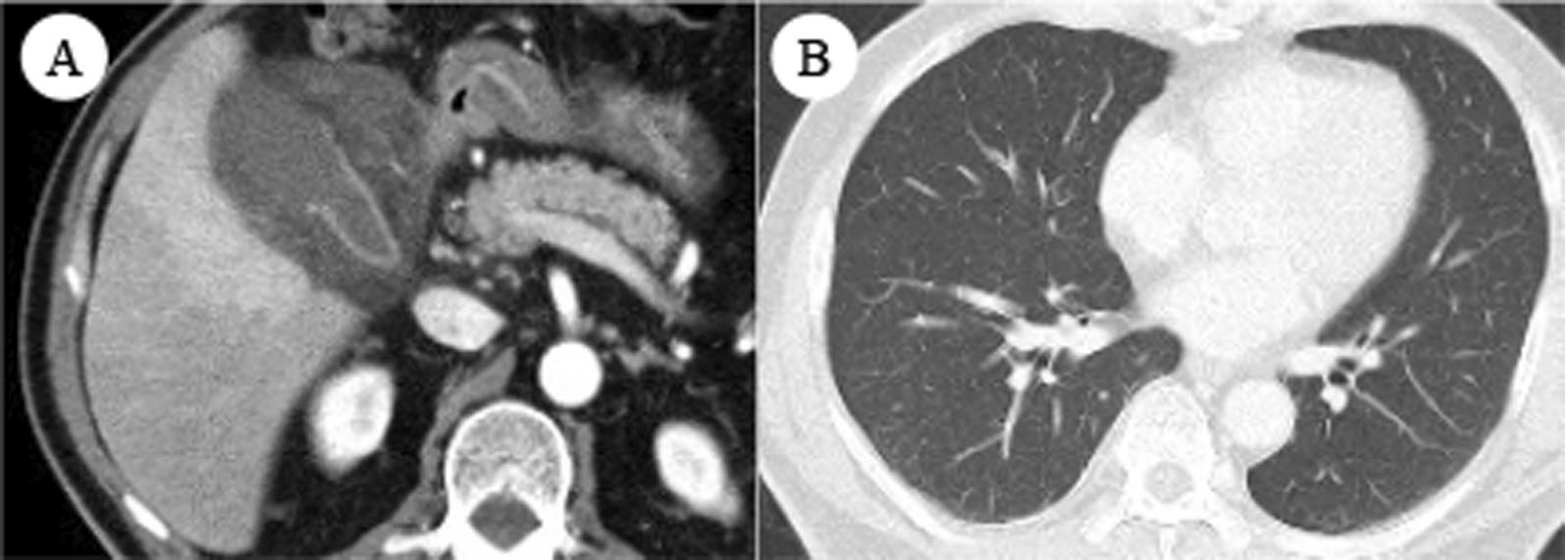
A Contrast-enhanced computed tomography revealed specific findings of gangrenous cholecystitis, including intraluminal membranes, an irregular or absent wall, and abscess formation. B Chest computed tomography showed no evidence of pneumonia
